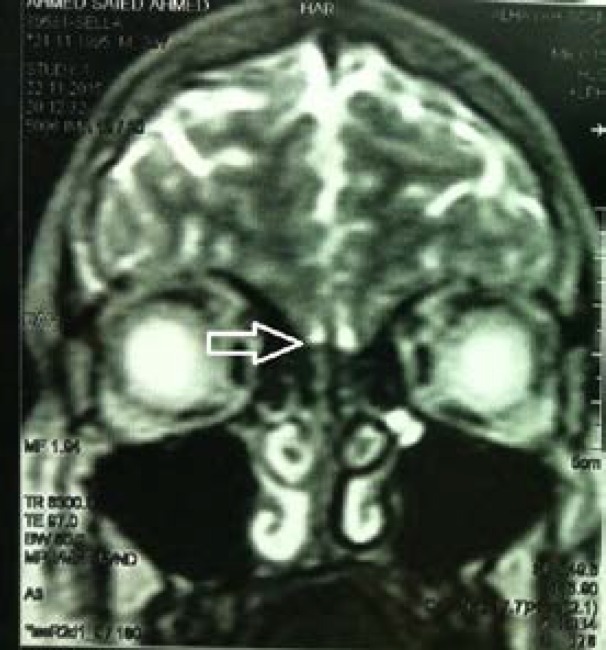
MRI scan of the brain identifying deficiency of the olfactory bulbs; the arrow denotes the presumed location of the olfactory bulbs
